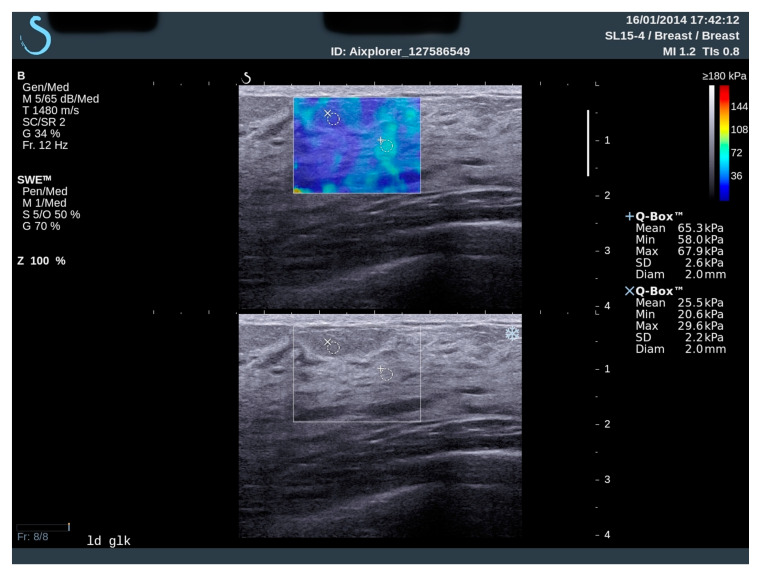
Elastography measurement. Region of interest is placed into representative place for fatty and glandular breast tissue. Ultrasound device shows elasticity values in color and kPa.
